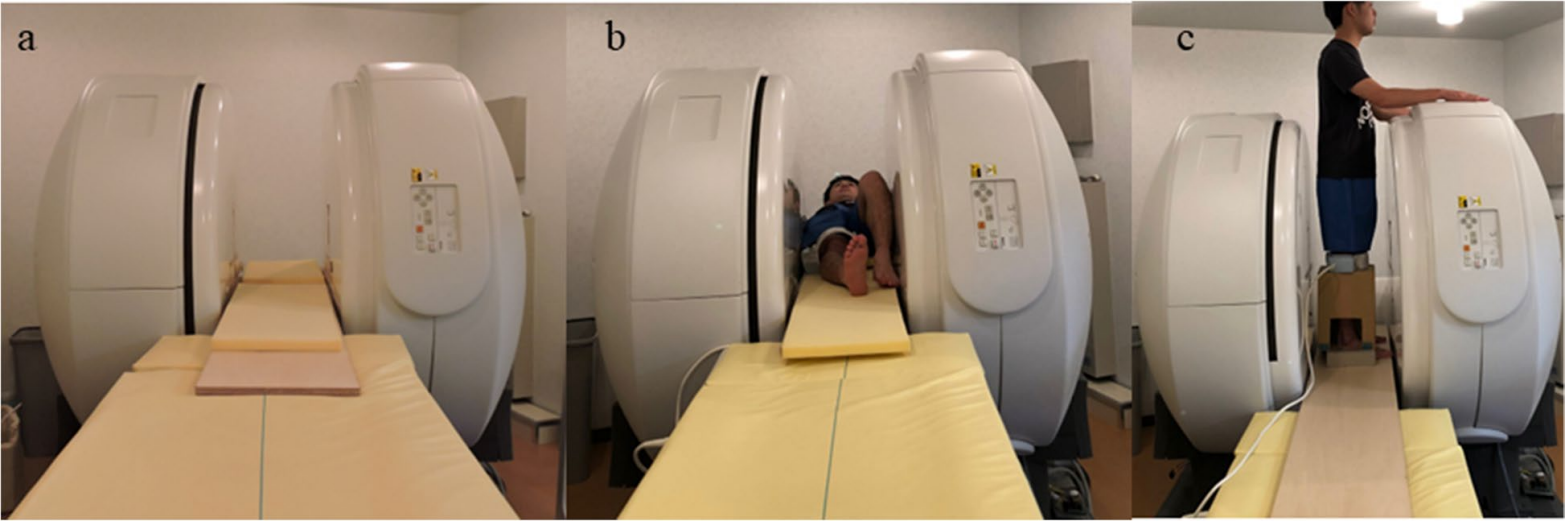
Process of magnetic resonance image capture: (a) MRI apparatus, (b) supine position, and (c) upright position. MRI, magnetic resonance imaging
